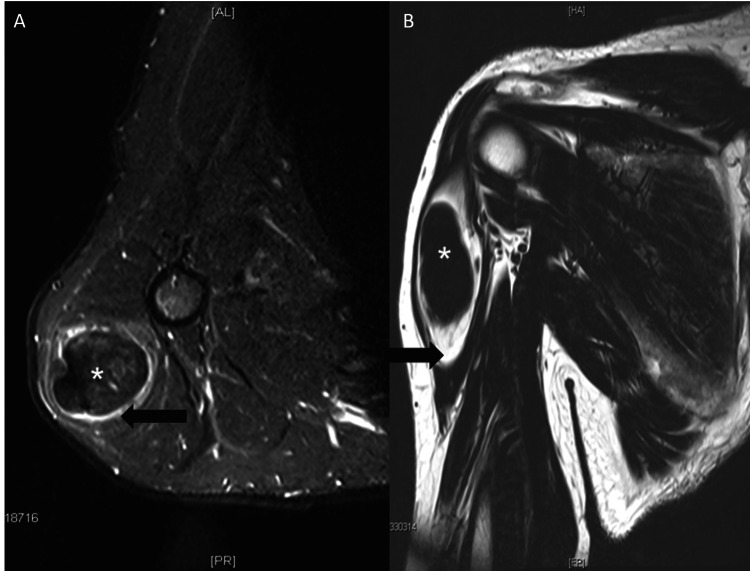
A) Axial T2 and B) coronal T1 MRI of the right shoulder girdle showing extra-abdominal fibromatosis (*) inside a lipomatous tumor (arrow) in the deltoid muscle.
